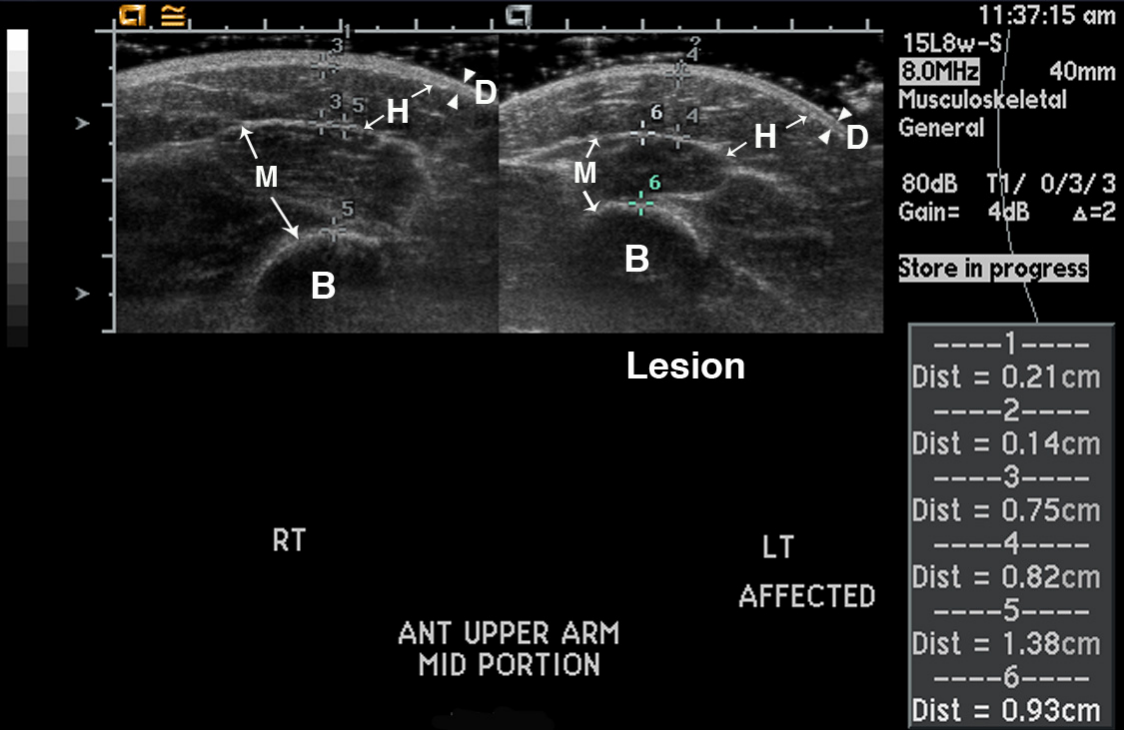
14 year old girl with linear scleroderma affecting her left arm; portion shown is from her upper arm. She has chronic atrophy from long standing disease (11 years), with persistent signs of disease activity including erythema and pruritis. Ultrasound shows varying patterns of echogenicity differences in the lesion, with no difference found in the dermis (dermis echogenicity = 0), mildly increased echogenicity in the hypodermis in a patchy pattern (hypodermis echogenicity = 1), and decreased echogenicity in the muscle (deep tissue echogenicity = -1). Ultrasound allows facile measurement of tissue thickness. Cursors were placed at tissue boundaries, using the highest point of the humeral bone as a landmark for the measurements. The lesion dermis is thinner than the normal dermis (measurement 2 (0.14 cm) vs 1 (0.21 cm), respectively; dermis tissue thickness score 1). The lesion hypodermis is mildly thicker than the normal hypodermis (measurement 4 (0.82 cm) vs 3 (0.75 cm), respectively; hypodermis tissue thickness score -1). The lesion muscle is thinner than the normal muscle (measurement 6 (0.93 cm) vs 5 (1.38 cm), respectively; deep tissue tissue thickness score 2). B = bone, D = dermis, H = hypodermis, and M = muscle. Arrowheads indicate boundaries of dermis, while arrows indicate boundaries of hypodermis and muscle. Large tick marks on x and y axis = 1 cm.
